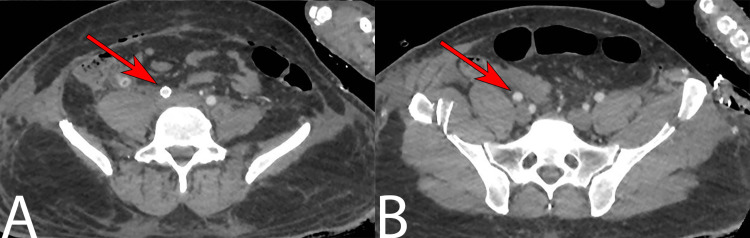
Axial CT images post-repair of the right common iliac artery. (A) Axial CT image demonstrating the stent that was placed in the right common iliac artery. (B) Axial CT image demonstrating patent right external and internal iliac arteries. CT: computed tomography
